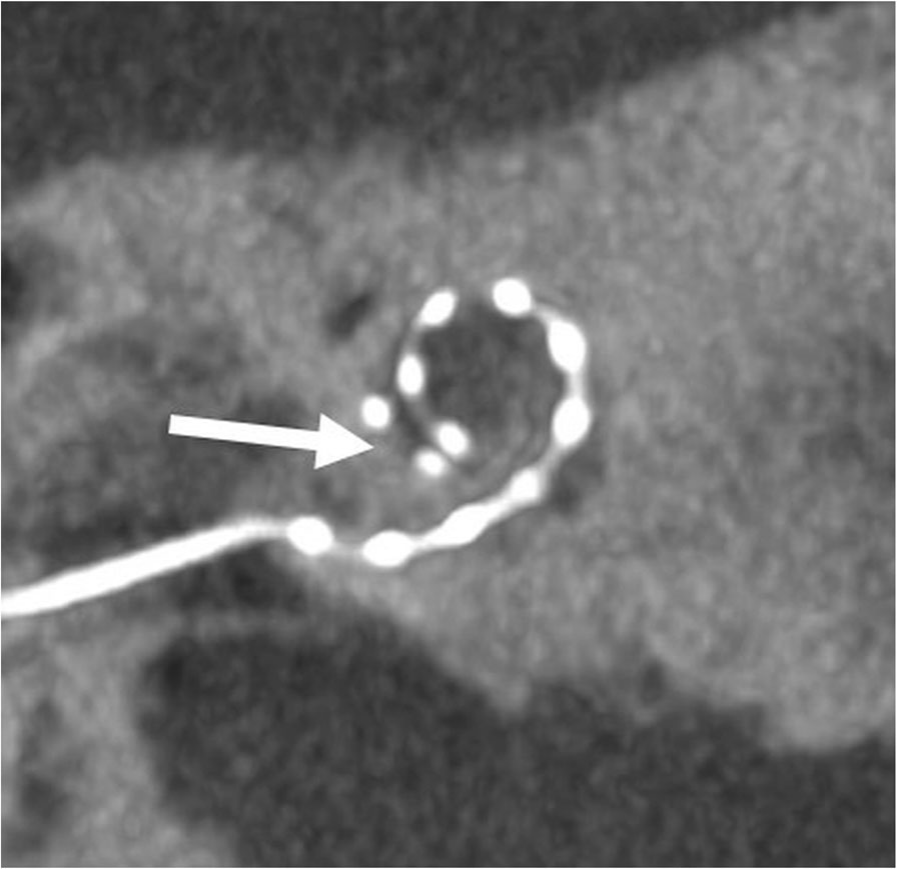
A 66-year-old male patient, with tip fold-over. CBCT paraaxial maximum intensity projection image shows fold-over of the tip of the electrode in the cochlea (arrow)
